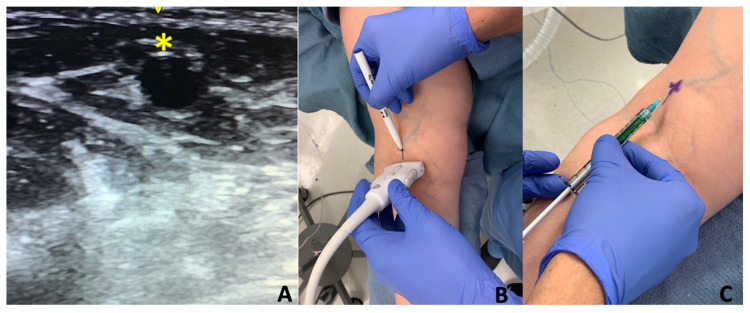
Preoperative ultrasound (US) localization over the cephalic vein. (A) The asterisk (*) demarcates the location of the cephalic vein using ultrasound technology. (B) Depiction of the operating surgeon mapping out the injection point 4 cm distal to the antecubital crease over the cephalic vein. (C) Targeted injection of ICG and albumin mixture to map the lymphatic anatomy of the upper extremity.
